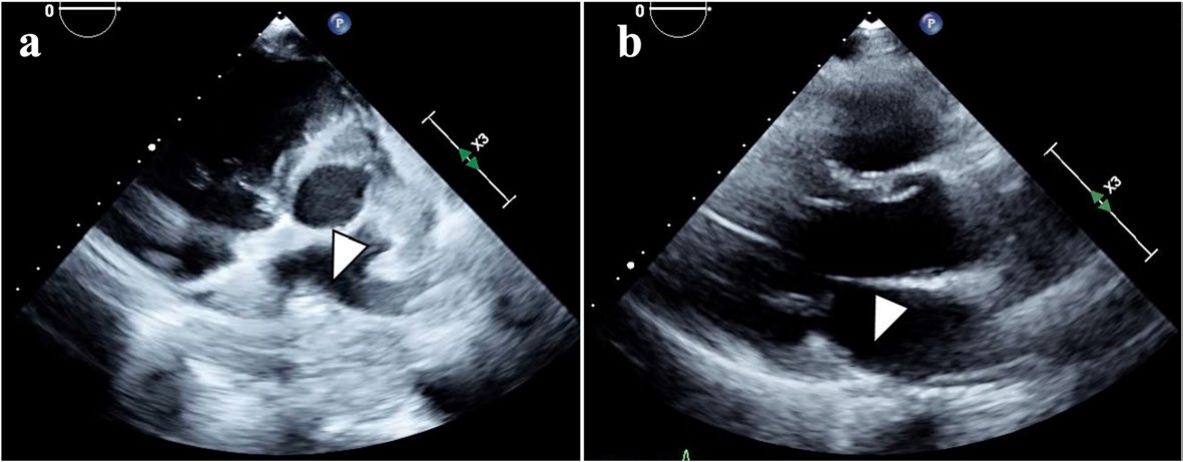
Echocardiography findings. a Initial echocardiogram showed hyperintense tumor invasion into the left atrium (white arrow head). b Echocardiography after four courses of chemotherapy showing no evidence of tumor invading the left atrium (white arrow head)
